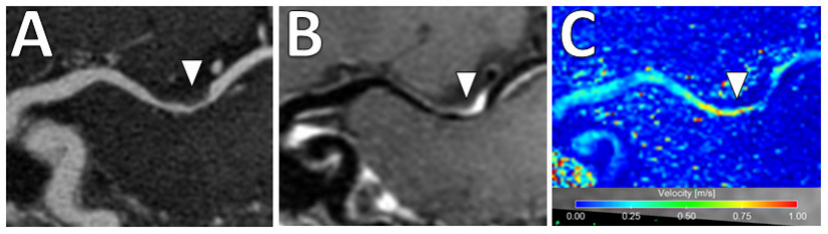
4D Flow MRI of intracranial atherosclerosis. (A) TOF MRA image demonstrates a focal area of middle cerebral artery narrowing (arrowhead) with (B) post-contrast T1-weighted VW-MRI showing a corresponding eccentric, enhancing atherosclerotic plaque (arrowhead). (C) On 4D Flow MRI, increased velocities are seen in the area of greatest narrowing (arrowhead).
